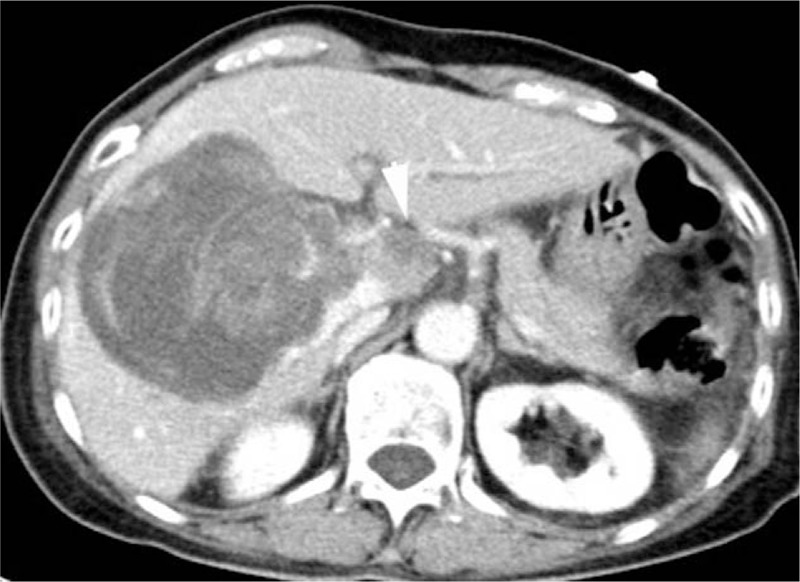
The computed tomography scan conducted after 2 months indicated a substantial increase in tumor size. The density of the tumor was uneven, and new lesions were discovered to be developing in the liver caudate lobe (arrow). The tumor invaded the blood vessels and bile ducts in the hepatic hilar region.
